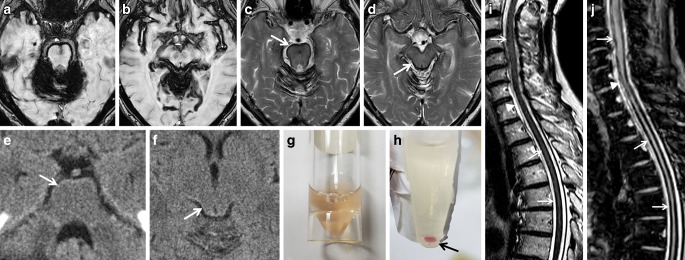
A 73-year-old woman suffering from recurrent severe headache attacks due to ventral dural defect at the level of the second thoracic vertebra with spontaneous intracranial hypotension and recurrent subarachnoid bleeding over more than 10 years. Axial susceptibility-weighted imaging (SWI) (a,b) and axial T2-weighted images (WI) (c,d; *arrow*) showing extensive superficial siderosis (SS) especially infratentorial; e, f CT disclosing slight hyperdense pontine and mesencephalic surface (*arrow*). Cerebrospinal fluid (CSF) analysis demonstrating auburn liquor (g); h xanthochrome supernatant and sedimentation of erythrocytes after centrifugation (h, *arrow*). i,j (SWI sag.) Extensive spinal SS (*arrows*) and ventral epidural fluid collection at the upper thoracic level (*arrowheads*)
